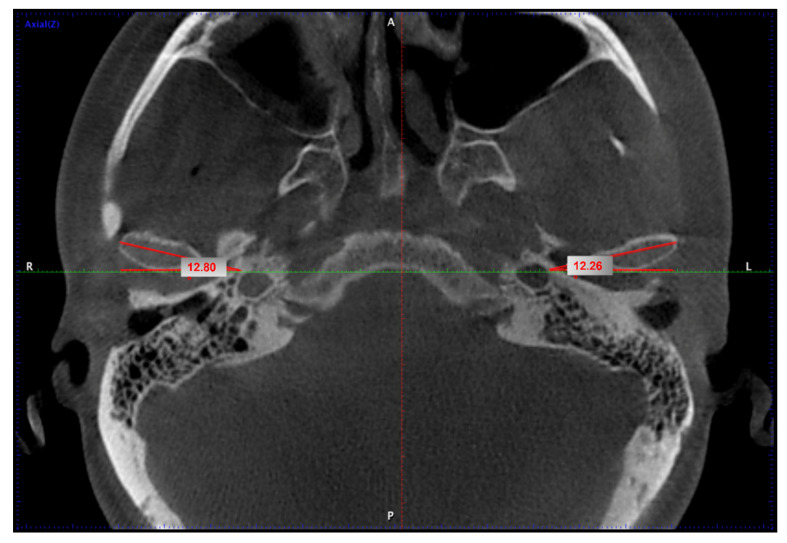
CBCT axial section. The measurements of the condyle angle performed in its largest diameter related to the transversal plane.
